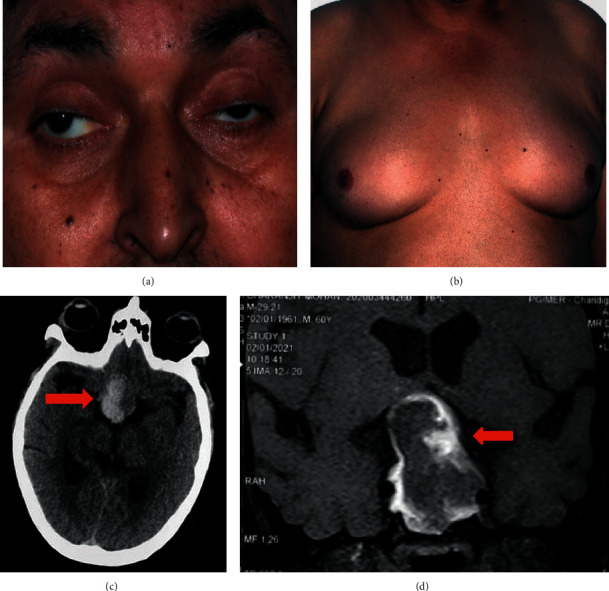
A 60-year-old male presented with sudden-onset severe headache and vision loss. (a) Bilateral ptosis and abducted right eye; (b) bilateral gynaecomastia; (c) axial NCCT head demonstrating a large sellar mass with areas of hyperdensity within suggestive of acute hemorrhage; and (d) coronal noncontrast T1-weighted MRI sellar demonstrating a 3.1 × 2.7 × 4.1 cm pituitary adenoma with peripheral T1 hyperintensity suggestive of acute bleed.
